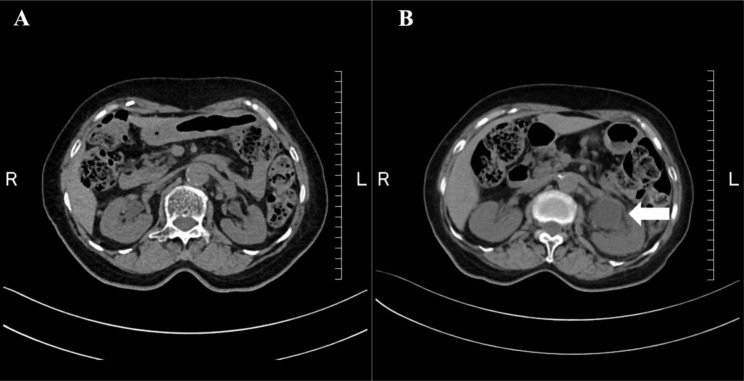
(A) An abdominal CT taken one year before admission revealed mild dilation of the left renal pelvis. (B) An abdominal CT taken on admission revealed dilation of the left renal pelvis (arrow) with an obstruction of unknown origin, and perinephric stranding of the left renal pelvis was observed CT, computed tomography
